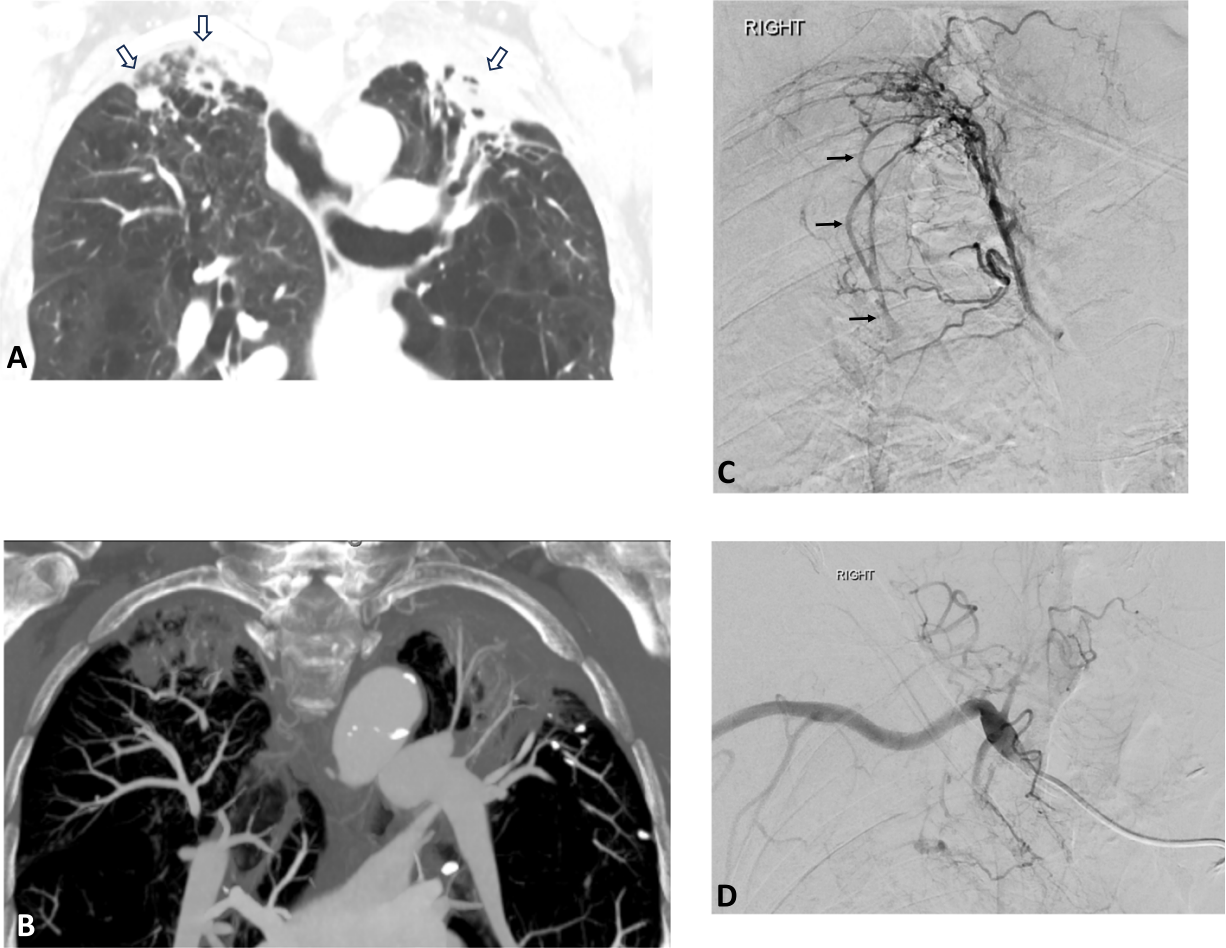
72-year-old woman with post-primary TB presenting with worsening haemoptysis. A Coronal CT image of the upper zones shows bilateral fibrotic changes and apical cavitations (open arrows). B Maximum intensity projection coronal CT image shows abnormal vessel recruitment by the apical inflammatory mycetomas. C Selective angiogram of the R intercostobronchial trunk shows recruitment of multiple intercostal arteries and florid neo-angiogenesis. The black arrows represent intercostal artery-to-pulmonary venous shunting. D Selective right subclavian angiogram demonstrates further vessel recruitment to the apical cavity
